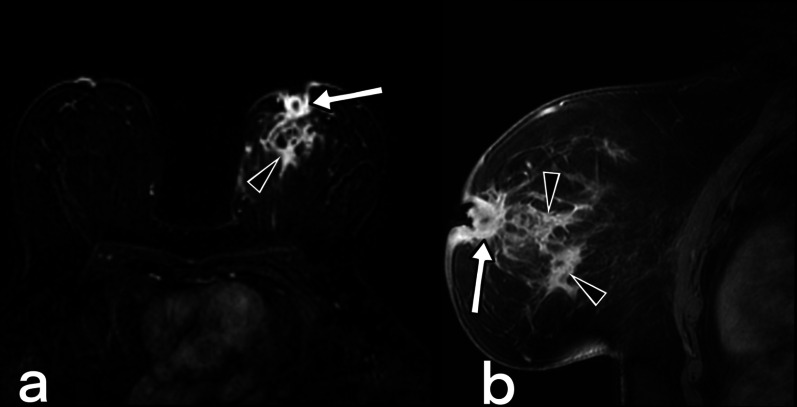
MRI images of a 37-year-old female. Axial (a) and sagittal (b) fat-suppressed T1 weighted contrast-enhanced images demonstrate nodular tuberculosis in the left breast. MR image shows an irregular shaped spiculated margins mass (arrowheads) with heterogeneous internal enhancement. The lesion mimics a breast carcinoma with nipple retraction (arrows). BIRADS 5
